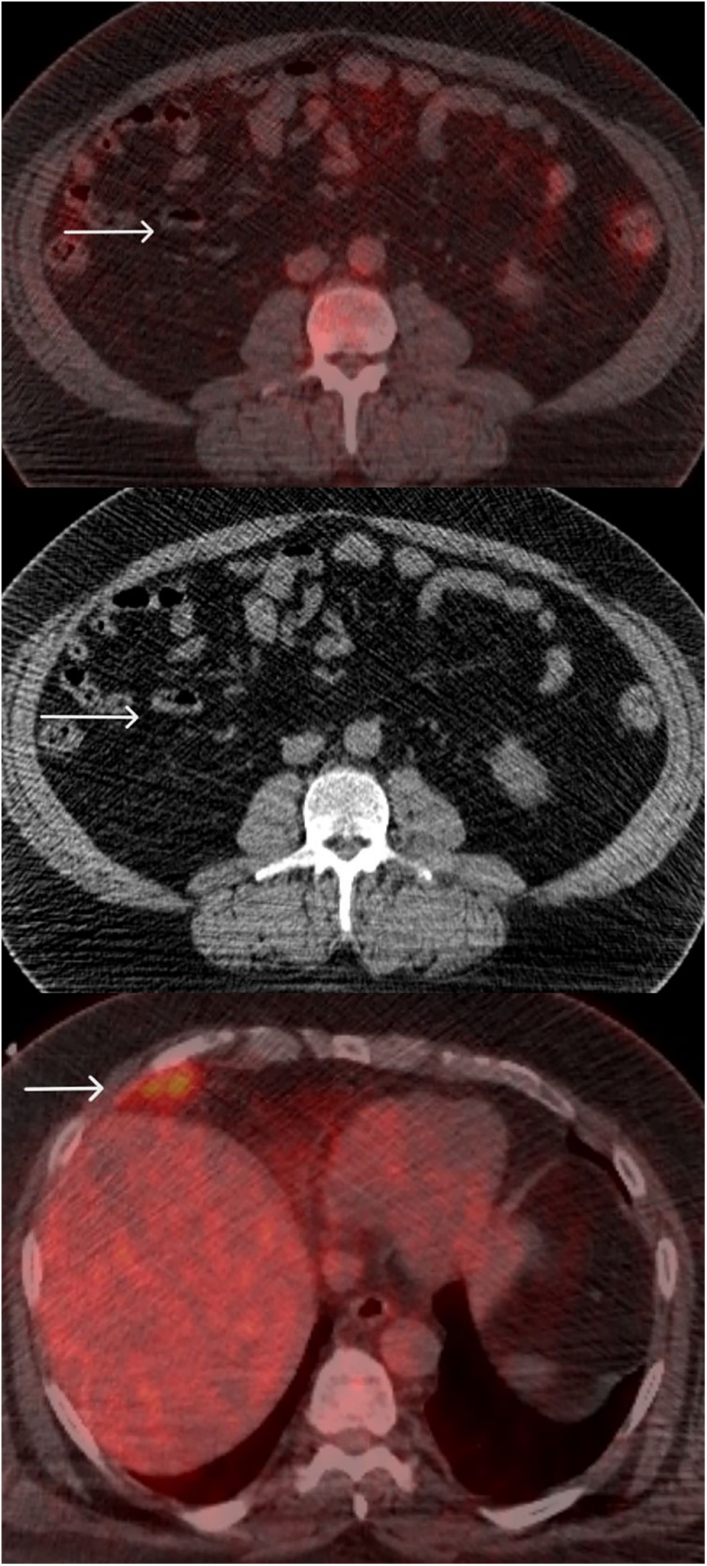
FDG PET/CT June 2016: follow-up PET/CT after 7 cycles of immunotherapy shows a positive response in the mesenteric node (Top image), but the perihepatic lesion is morphologically stable. The FDG uptake reduced from SUV max 6.5 to 5.3 (background hepatic SUV max 4).
